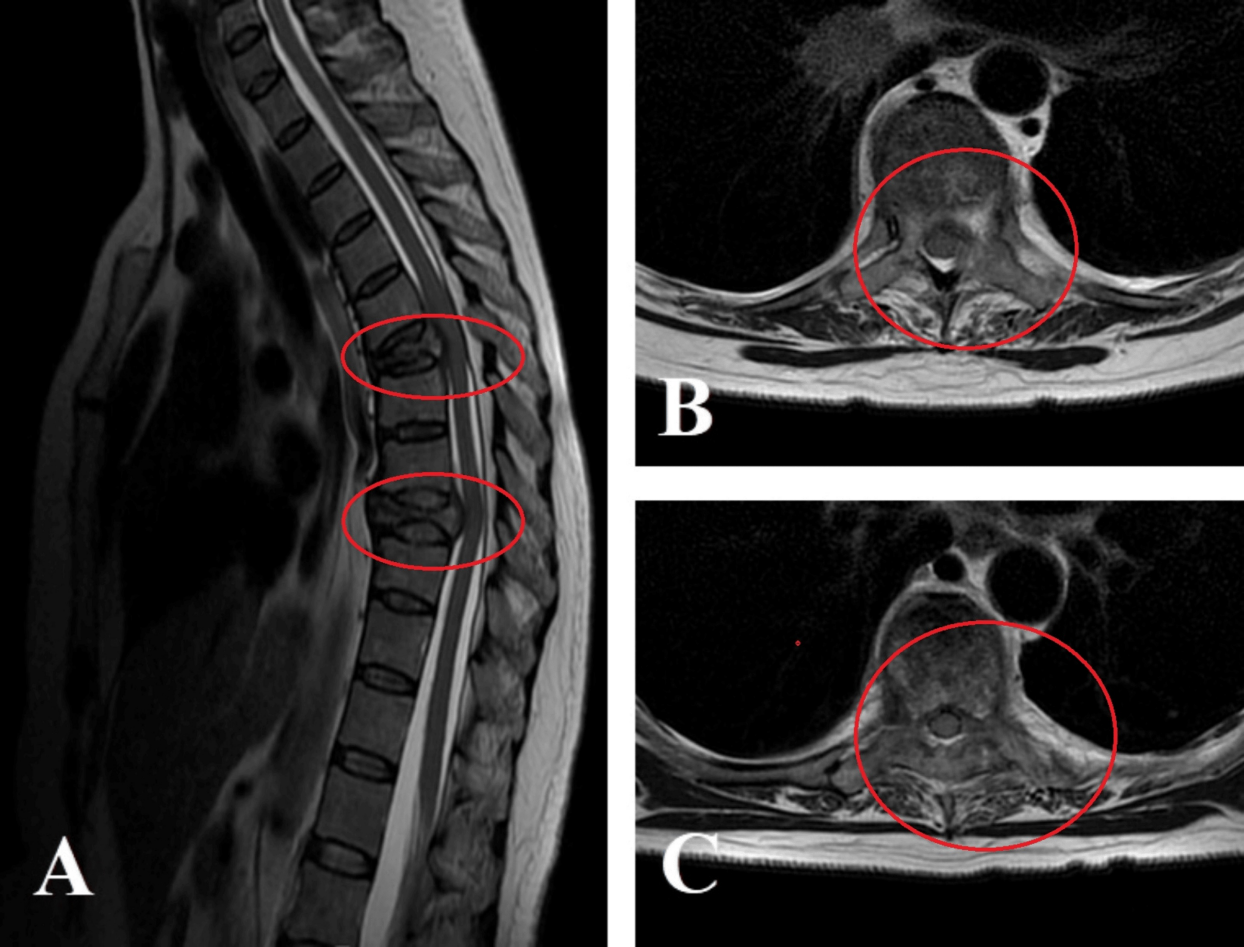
Sagittal (A) and axial (B, C) MRI scans of the thoracic spine show metastatic lesions at T6 and T9 with spinal cord compression, loss of more than 50% of body height, and invasion into the spinal canal. Mainly, ventral compression of the spinal cord is observed.
